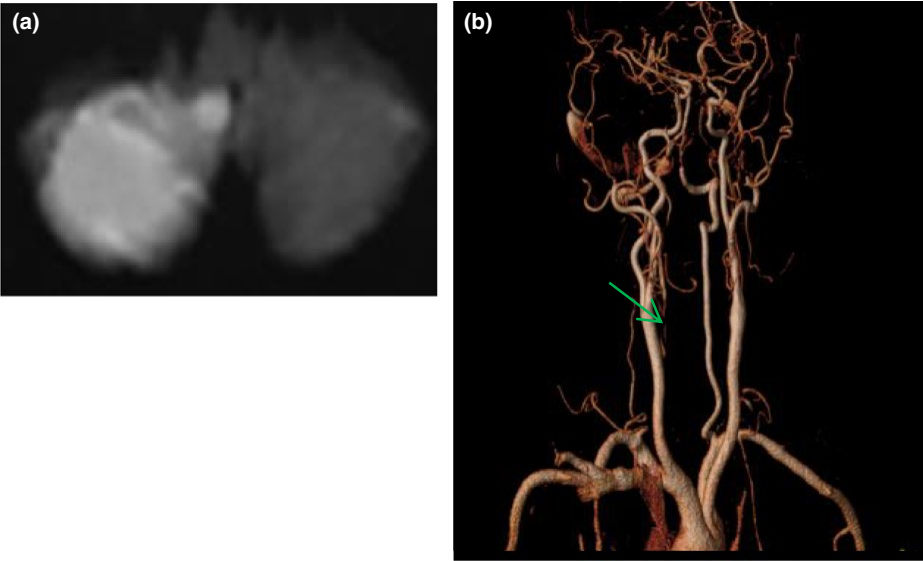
MR image of a 61‐year old man with acute vertigo. DWI showed acute infarction in right cerebellar hemisphere (a), neck vessel MRA showed proximal segment of right vertebral artery absent (green arrow, b)
